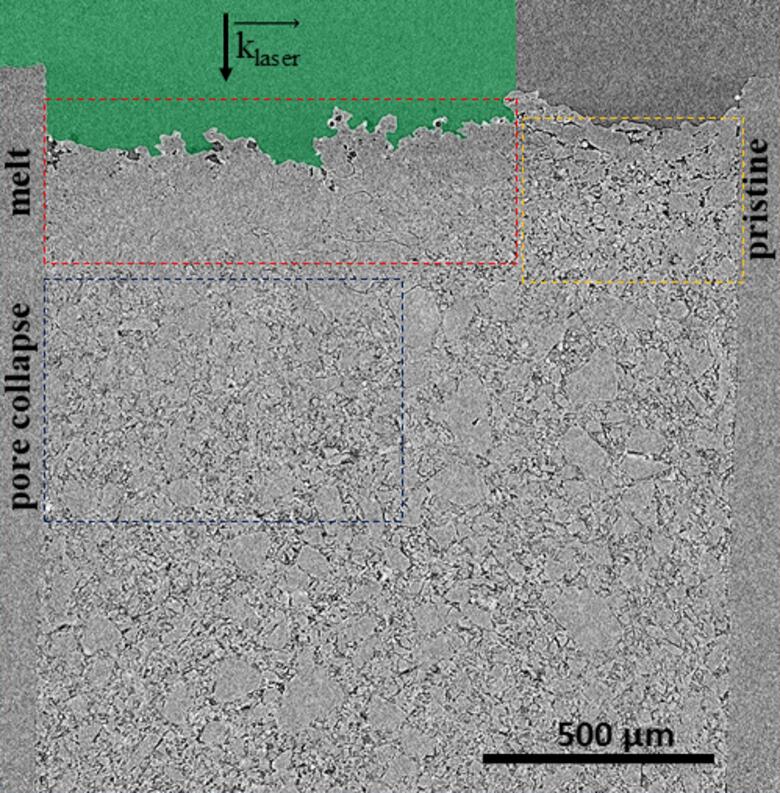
X-ray tomographic analysis of a shocked sample. The laser spot is position is added for reference. Below the laser-irradiated zone, a melted area is visible (red rectangle) as well as a pore collapse zone (blue rectangle). A non-irradiated zone is highlighted for reference (yellow rectangle).
